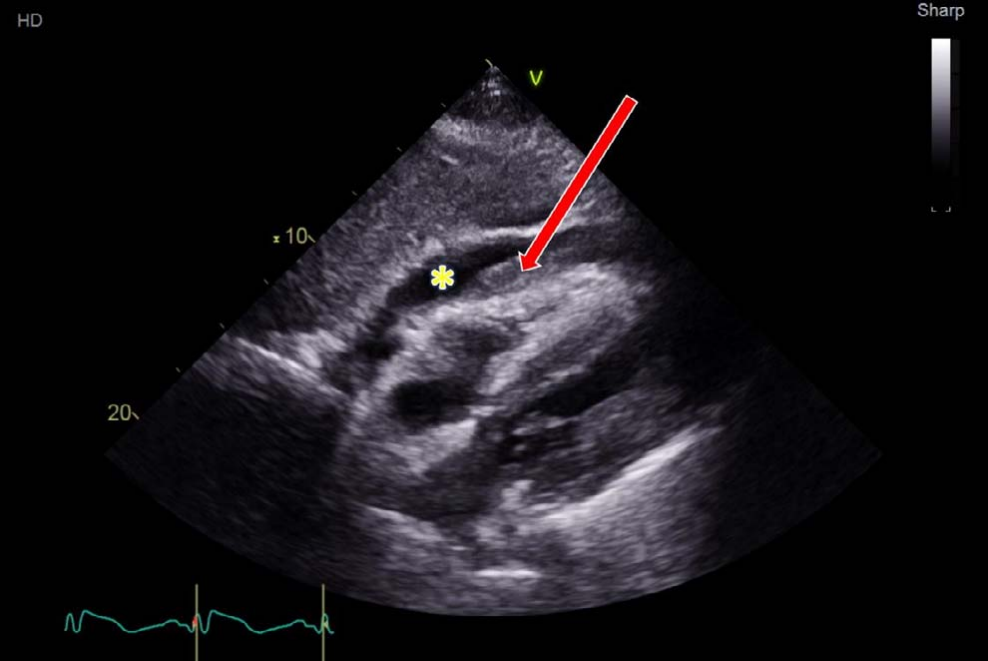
Transthoracic echocardiogram in subcostal view. The yellow asterisk denotes the pericardial effusion, and the red arrow points to echo-dense coagulum within the pericardial effusion.
